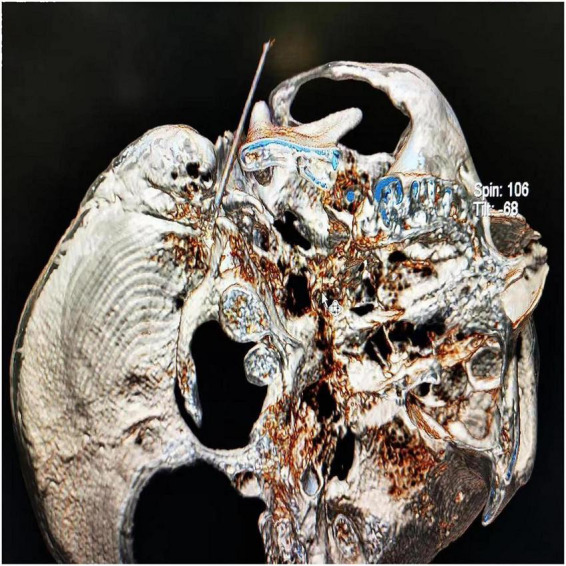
Three-dimensional reconstruction of the CT scans confirmed successful puncture of the stylomastoid foramen.
